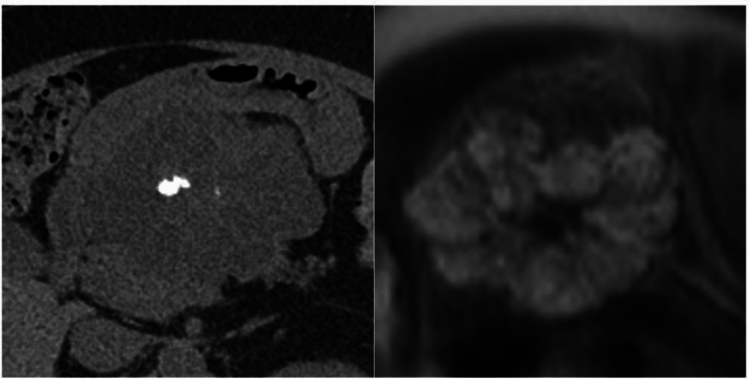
57-year-old woman with serous cystadenoma. a CT image showing a coarse internal calcifictaions (type 3 pattern); b on MR, the lesion presents the typical “honeycomb” appearance, with T2-hyperintense content
